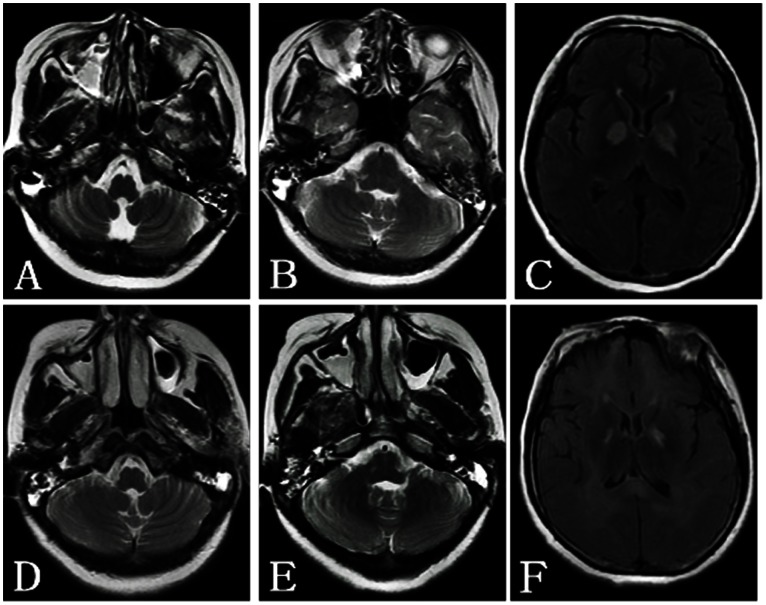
A 41-year-old woman with barotrauma of the bilateral middle ears and right maxillary sinus. A–B: The bilateral mastoid cavities, left middle ear cavity and right maxillary sinus show hyperintensity on T2WI. C: The bilateral globus pallidus shows symmetric hyperintensity on T2WI FLAIR. D-E: Two months later, the left maxillary sinuses, and the sphenoid sinus besides the sites above show hyperintensity on T2WI. F: The symmetric hyperintensity can be observed in the bilateral globus pallidus and the bilateral white matter on T2WI FLAIR.
